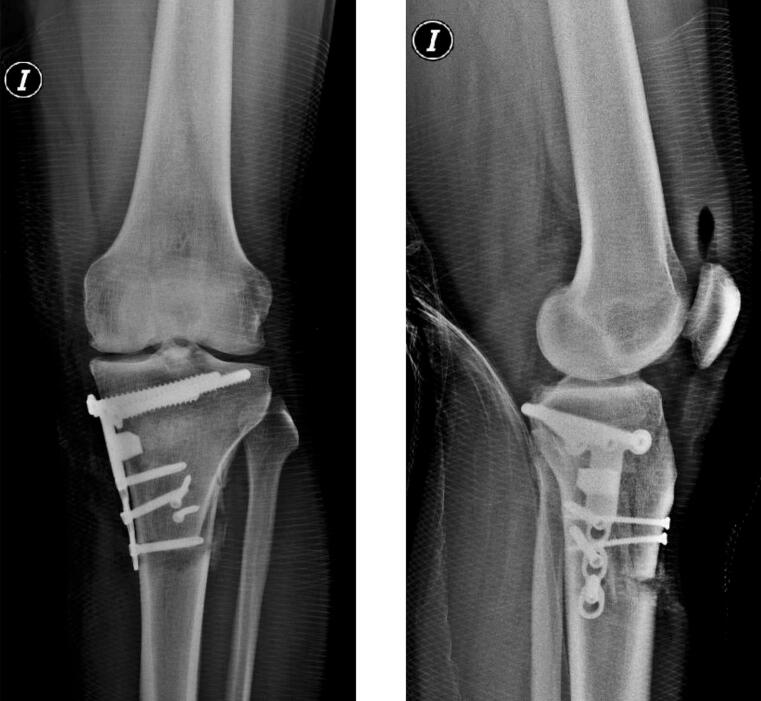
Postoperative radiographic follow-up of the “L”-shaped tibial osteotomy.
